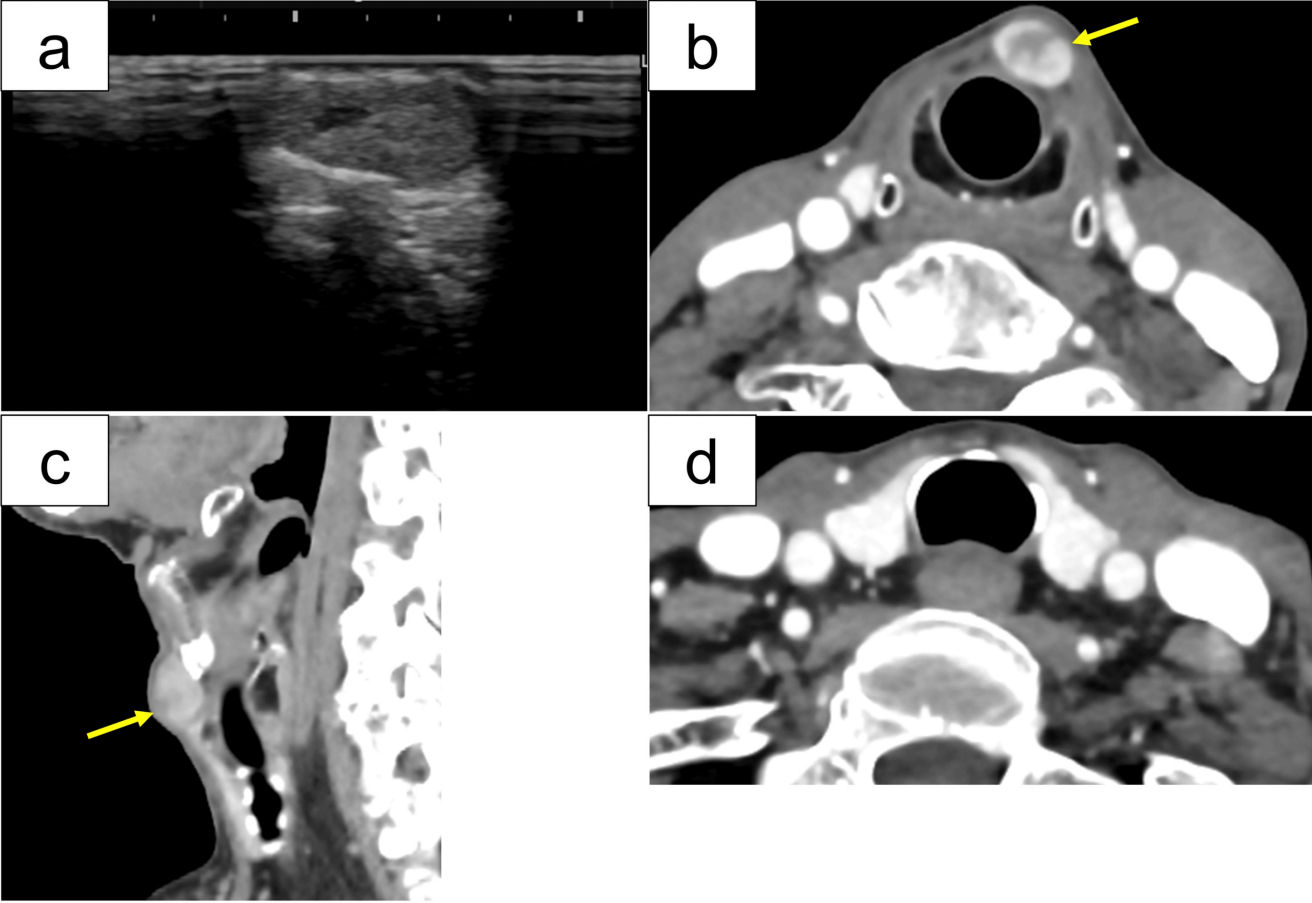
Preoperative imaging findings (a) Ultrasonography reveals a well-defined hypoechoic lesion with solid components and no internal calcification. (b, c) Axial (b) and sagittal (c) computed tomography (CT) images show a 10 × 10 mm mass located just superior to the thyroid cartilage with solid content (arrow). (d) Additional axial CT image confirming that the orthotopic thyroid gland is in the normal position without abnormalities.
